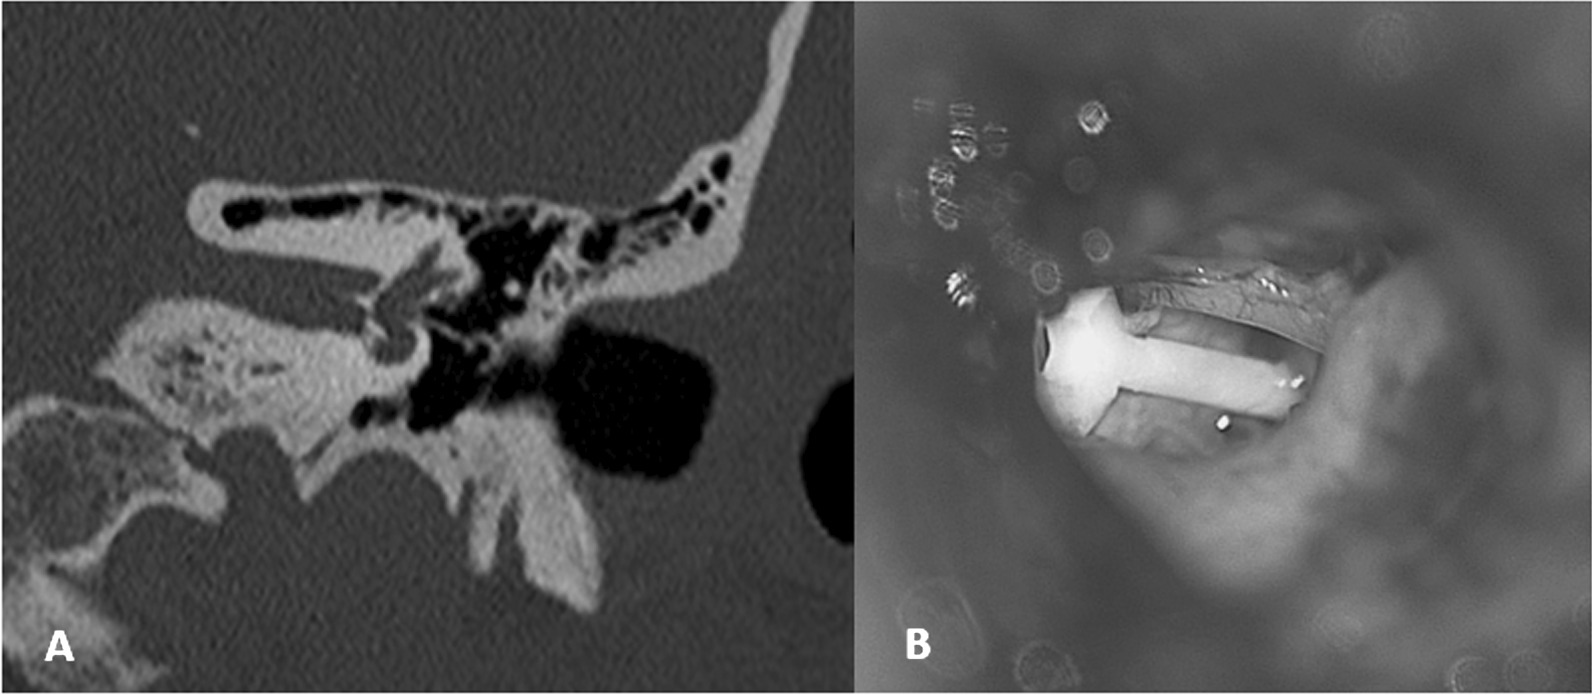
A Left ear CT-scan with coronal reconstruction in the axis of a dislocated prosthesis. B Per-operative view of a dislocated piston during revision stapes surgery of a left ear
